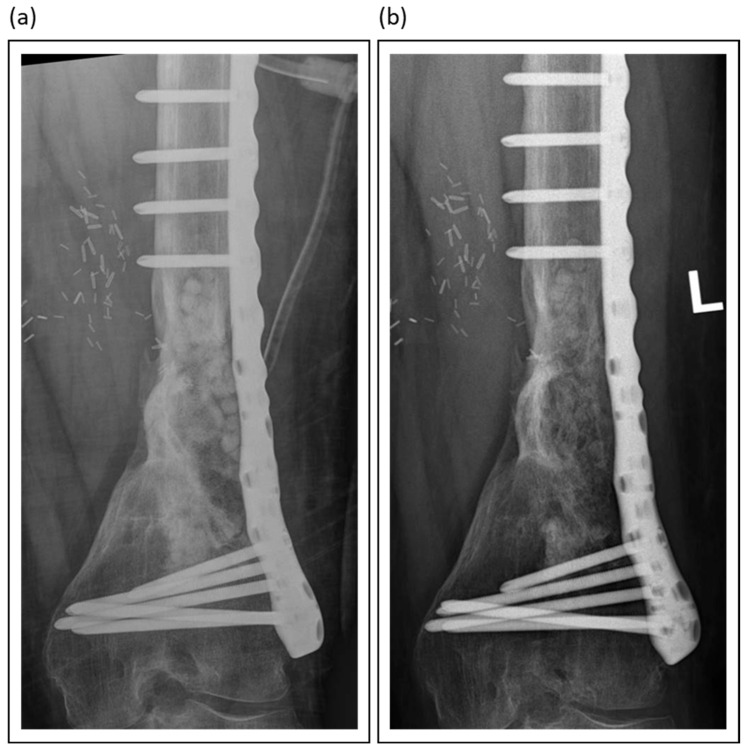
PerOssal^®^ as part of the treatment of chronic osteomyelitis in a distal femur: (a) Postoperative X-Ray image; (b) Follow-up X-Ray 2 years later. The PerOssal^®^-Beads are largely resorbed. No recurrence of infection was detected clinically.
